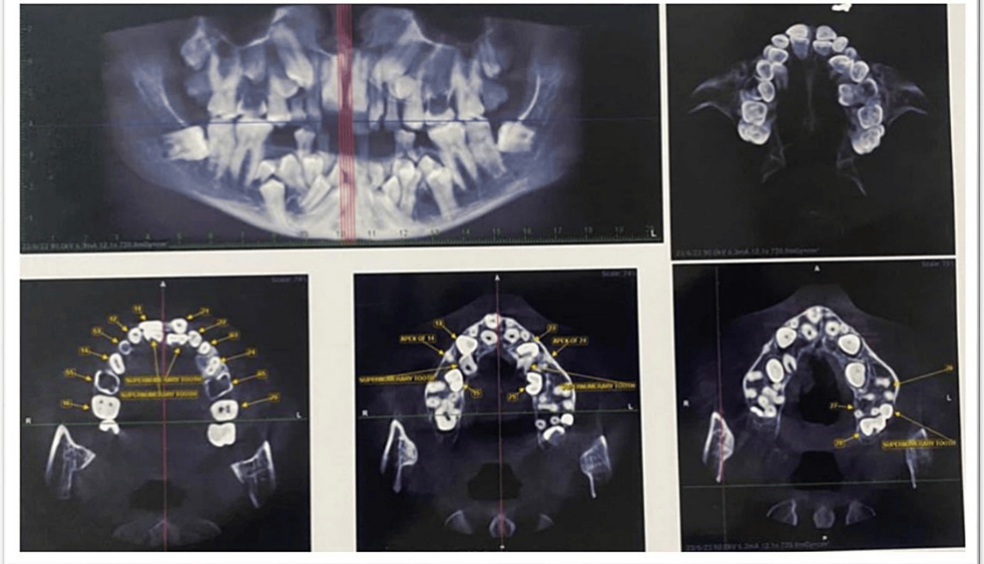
CBCT images CBCT: cone beam CT
